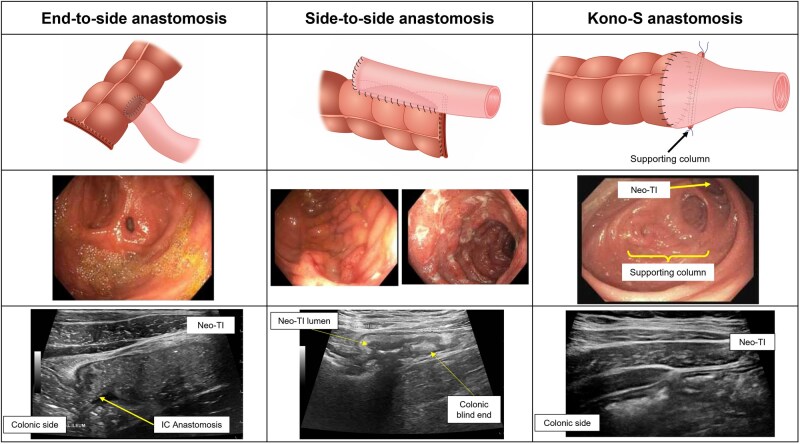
Ileocolic anastomosis configurations with corresponding appearance on endoscopy and intestinal ultrasound. IC, Ileocolic anastomosis; Neo-TI, neoterminal ileum.
